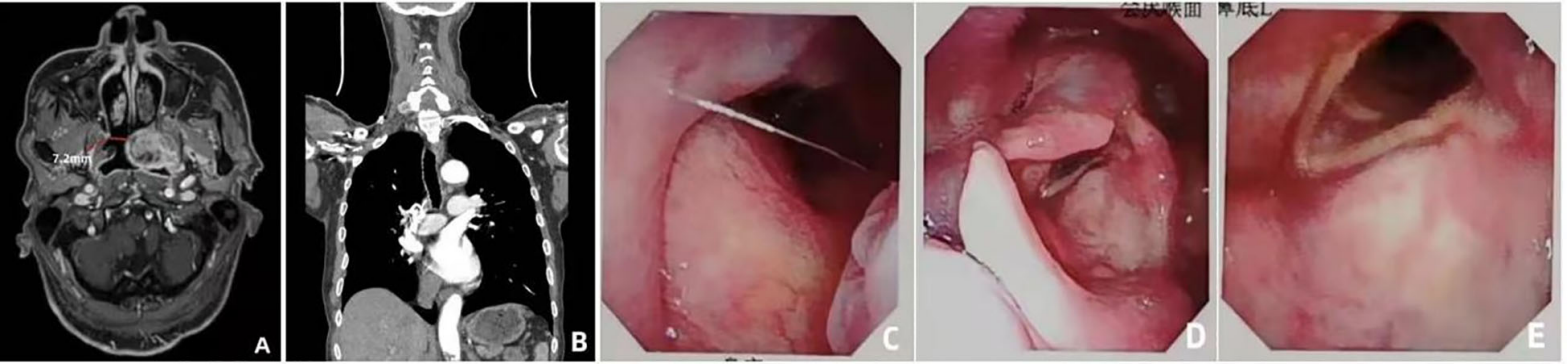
Endoscopic evaluation and airway assessment (A) Coronal CT showing stenosis at the right nasal-nasopharyngeal junction (narrowest diameter: 7.2 mm). (B) Sagittal CT showing normal structure of the larynx and lower airway. (C) Transnasal fiberoptic laryngoscopy showing a smooth-surfaced neoplastic bulge in the soft palate area. (D) Fiberoptic view of small smooth protrusions on the laryngeal surface of the epiglottis. (E) Fiberoptic view of symmetrical bilateral vocal cords with normal closure function.
